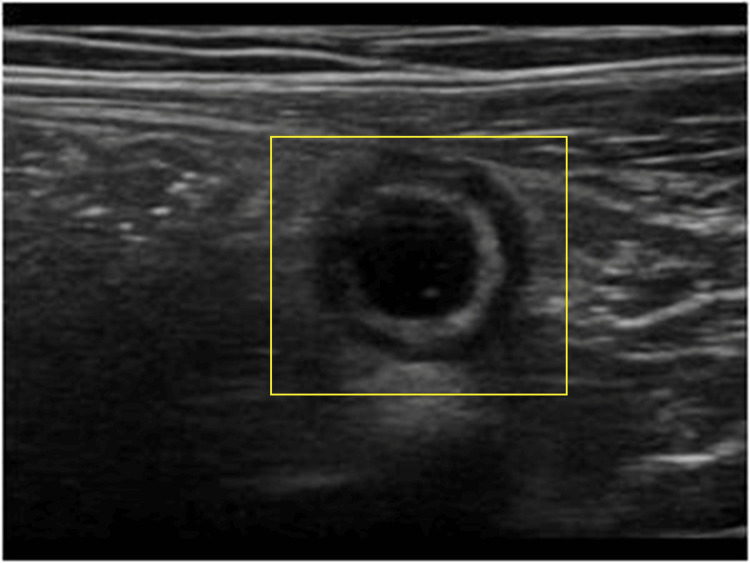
Ultrasound imaging of the abdomino-pelvic region Highlighted area indicates a heterogenous cystic mass lesion in the right iliac fossa
